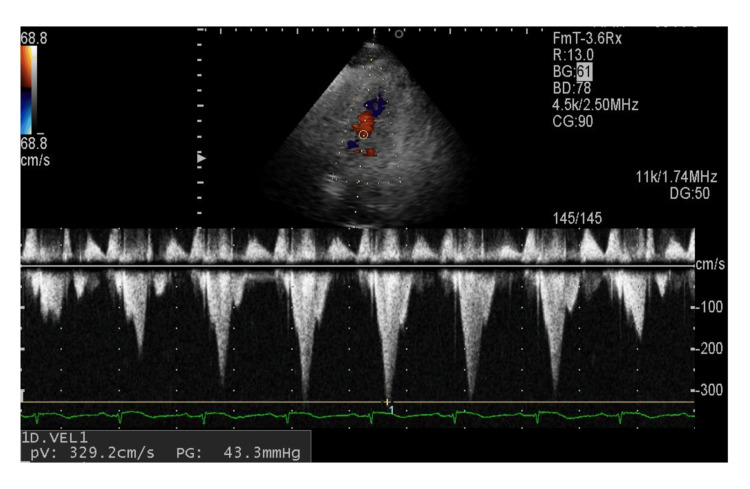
Doppler imaging in TTE findings. Continuous wave Doppler imaging in apical two-chamber view of TTE on day 14 showed a peaking dynamic pressure gradient of 43.3 mmHg in the left ventricular outflow tract. TTE: transthoracic echocardiogram
